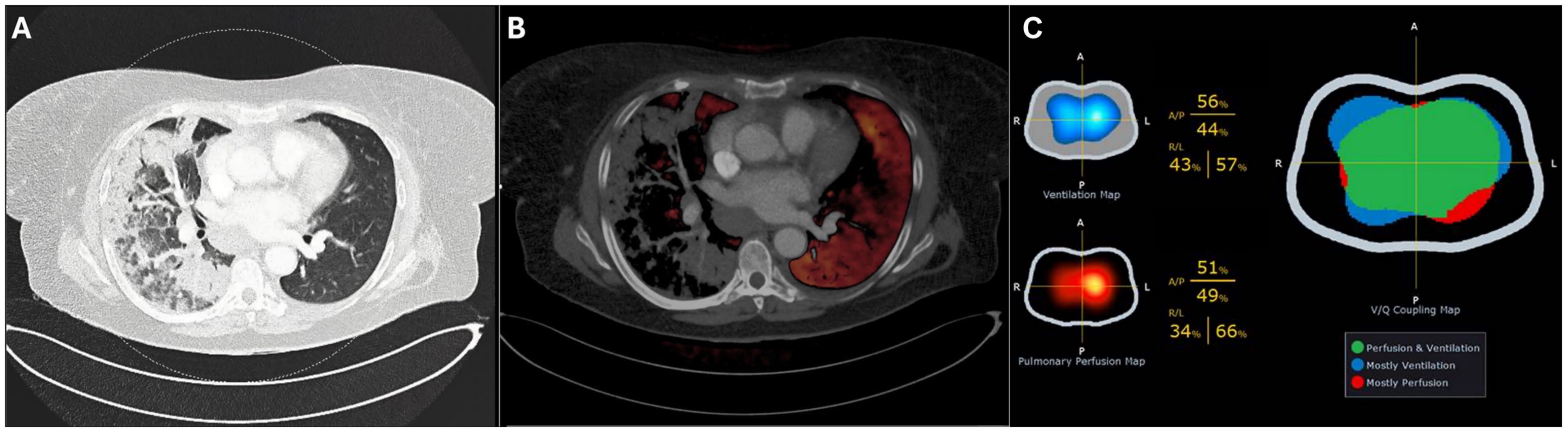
Dual-Energy CT in supine position showing the pulmonary window (A) and perfusion overlay (B). Electrical impedance tomography (EIT) on day 4 (C) shows the ventilation and perfusion maps (left) and the ventilation–perfusion (V/Q) map (right), where matched regions appear in green, predominantly ventilated areas in blue, and predominantly perfused areas in red.
